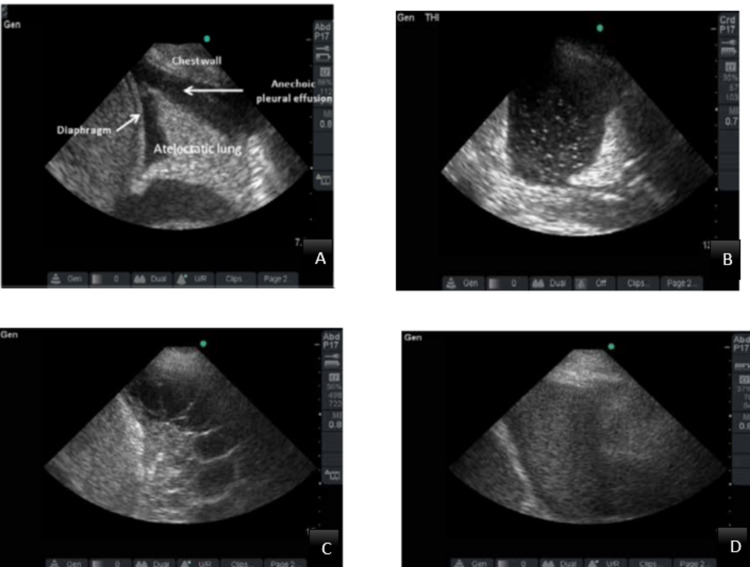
Ultrasound of lungs showing pleural effusion. (A) Pleural effusion is present as an anechoic space with anatomic boundaries made up of chest wall, diaphragm, and atelectatic lung. (B) Complex non-septated pleural effusion with swirling echogenic debris (Plankton Sign). (C) Complex septated pleural effusion. (D) Homogenously echogenic pleural effusion. Image courtesy by Rahul Khosla, Pulmonary & Critical Care Medicine, Veterans Affairs Medical Center, Washington, DC, USA, Lung Sonography chapter 6. Open access. Creative commons license.
